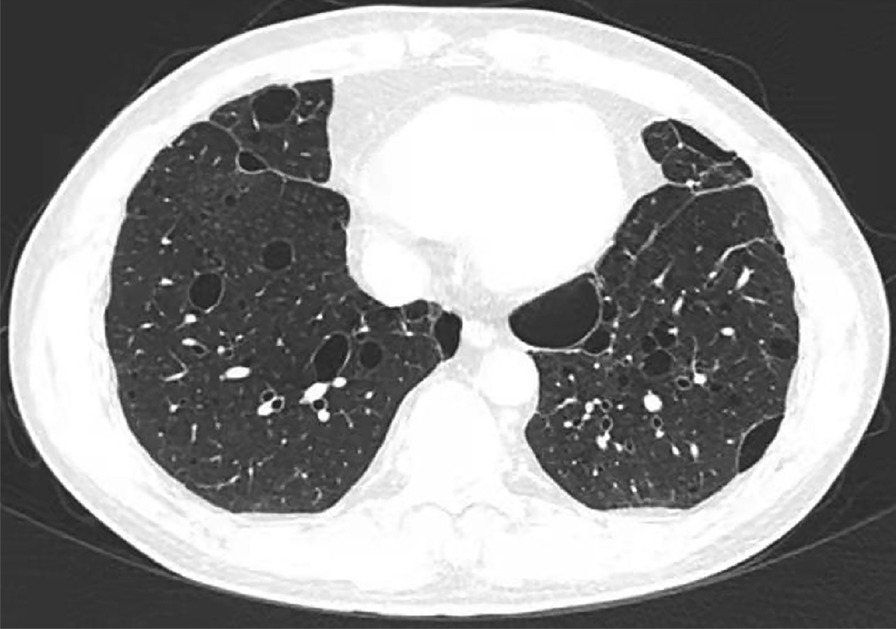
Chest high-resolution computed tomography scan showing multiple thin-walled cysts in bilateral lung and emphysematous bullae
